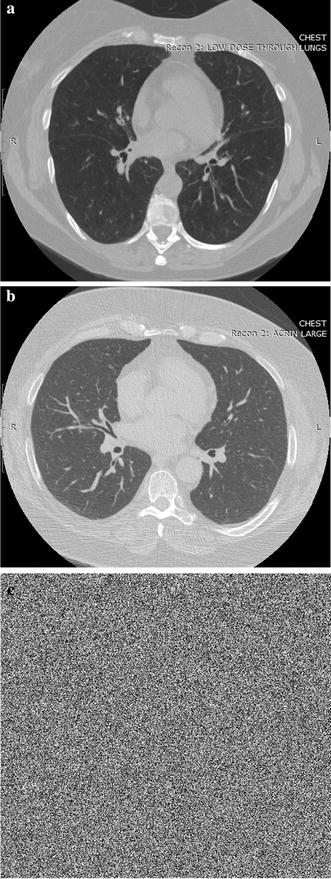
Decryption result of known-plaintext attack with the keys generated from a chosen CT sequence. a Original image. b Image used for attack. c Decrypted image with fake decryption keys
